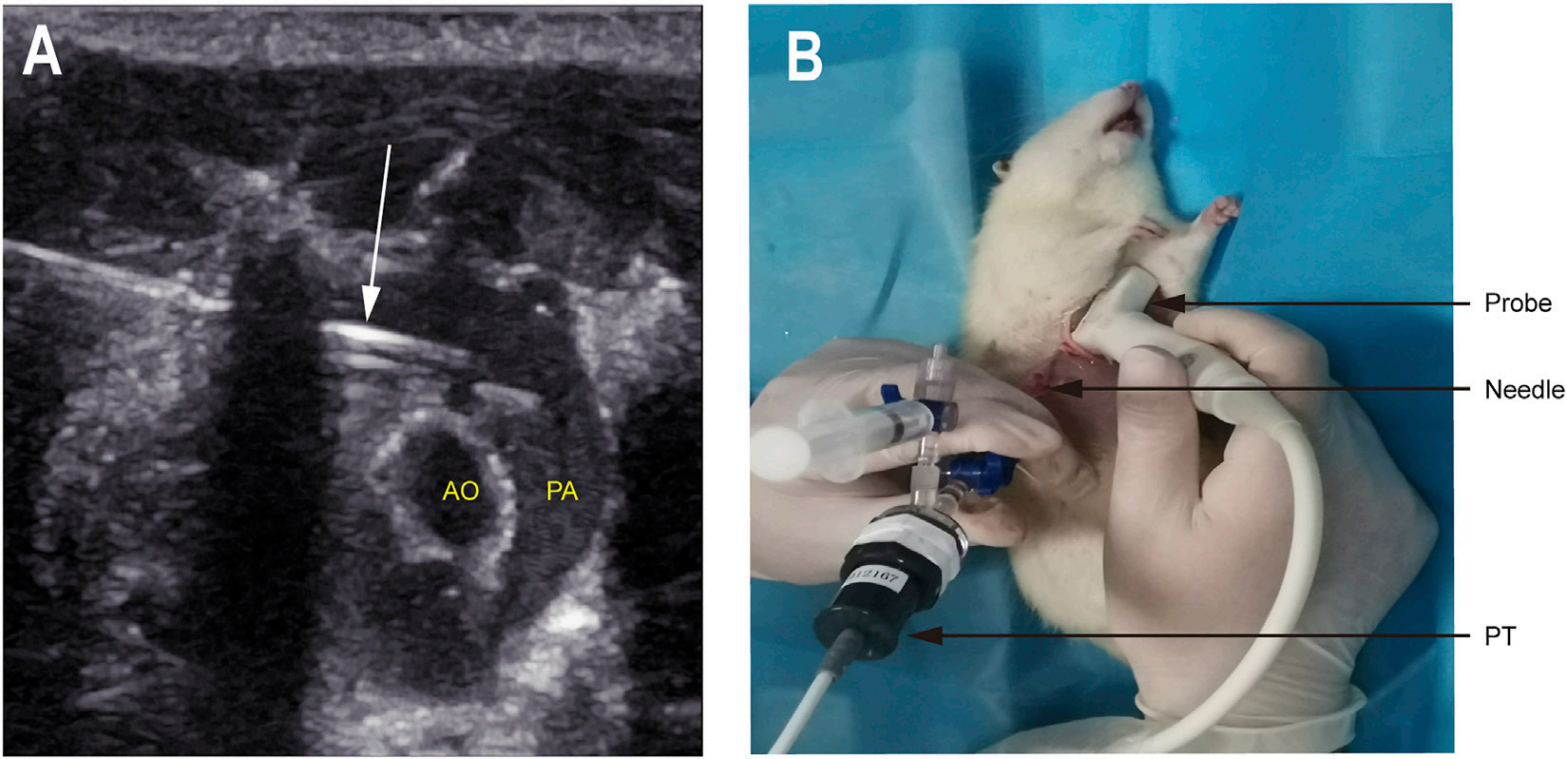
Echocardiography guided transthoracic puncture measurement of pulmonary artery pressure. (A) The puncture needle tip (white arrow) was located in the PA. (B) The actual operation of PA puncture by echocardiography was guided. Abbreviations: PA, pulmonary artery; AO, aorta; PT, pressure transducer.
